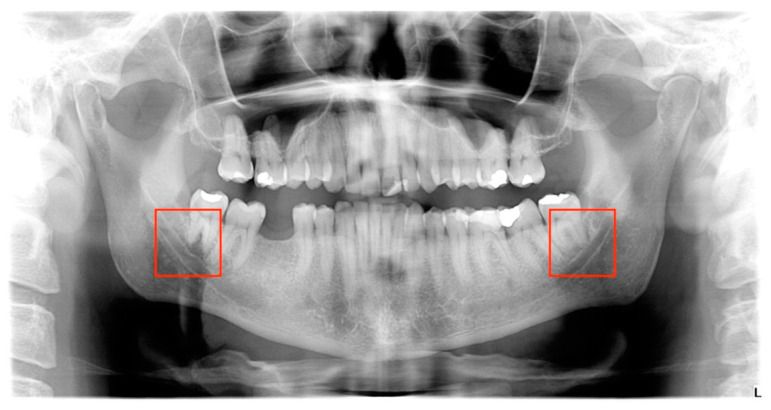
A panoramic radiograph with two red boxes designating the regions of interest (ROI). A location close to the canal and molar was chosen for the ROI’s center. The 2137 × 1024 pixel panoramic image was cropped to 224 × 224 pixels.
